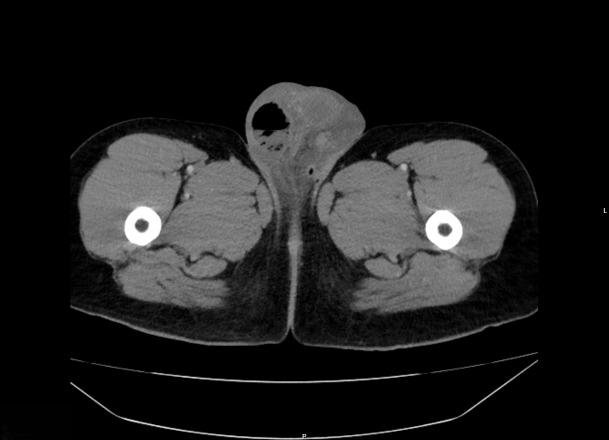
Computed tomography (CT) (transverse plane) of the abdomen and pelvis confirms the presence of gas and debris in the right side of the scrotum, including within the testicle and epididymis.
